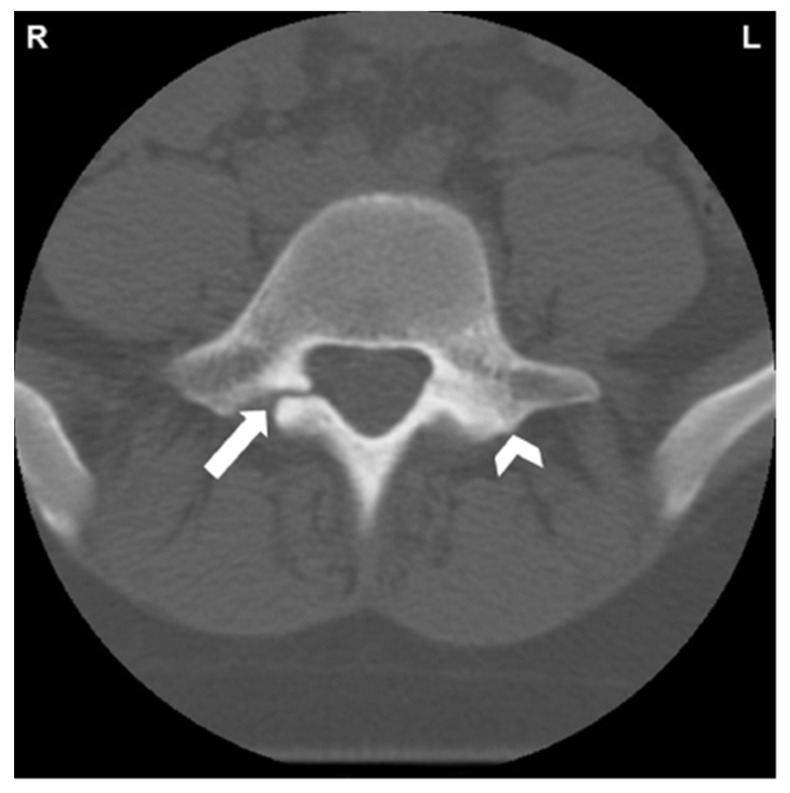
CT of L5 vertebral body and posterior elements. White arrow indicates spondylolysis of right pars interarticularis. Reactive sclerosis in the left pars demonstrated by white arrowhead [34]. “Reprinted from Neural arch bone marrow edema and spondylolysis in adolescent cheerleaders: A case series. *J. Chiropr. Med.* 2019, *18*, 335–342; with permission from Elsevier”.
